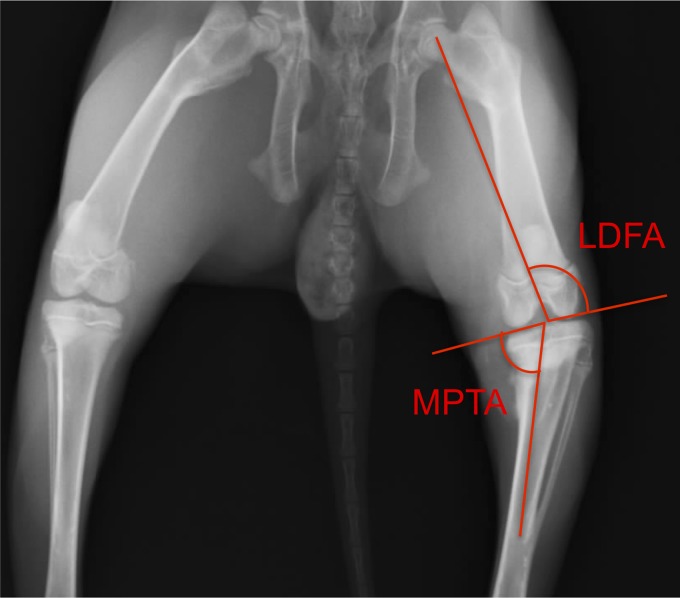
Medial proximal tibial angle (MPTA) and lateral distal femoral angle (LDFA) shown on radiograph.
